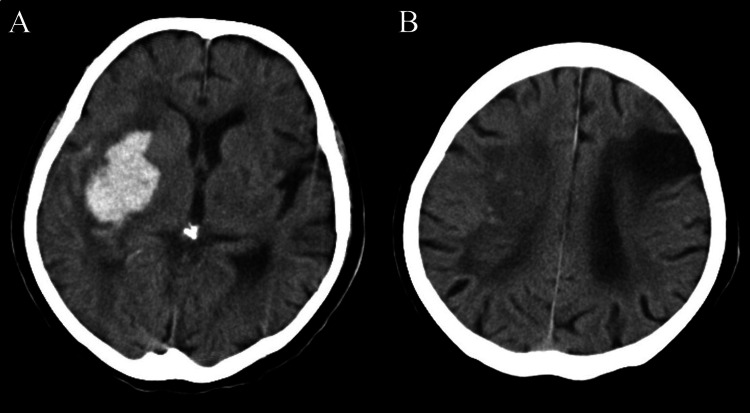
Initial head CT showing right putaminal hemorrhage (A) and chronic left cerebral infarction (B).
